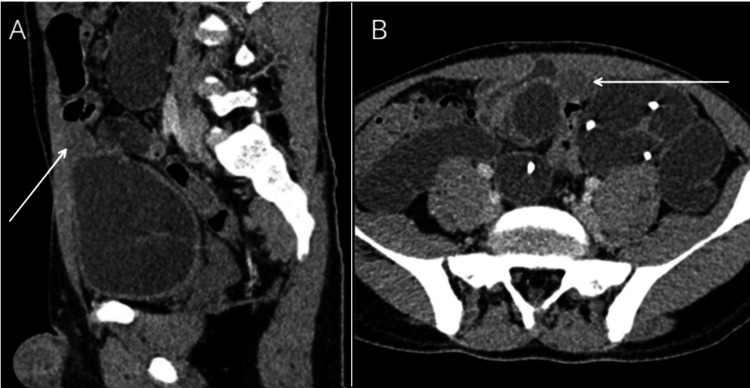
CT scan demonstrates a supravesical mass consistent with an ectopic testis. (A) Sagittal CT scan showing the ectopic testis located above and anterior to the urinary bladder, delineated as an oval soft-tissue density clearly separated from midline pelvic structures. (B) Axial CT image demonstrating the ectopic testis as a well-defined, oval soft-tissue mass situated supravesically. CT: computed tomography.
